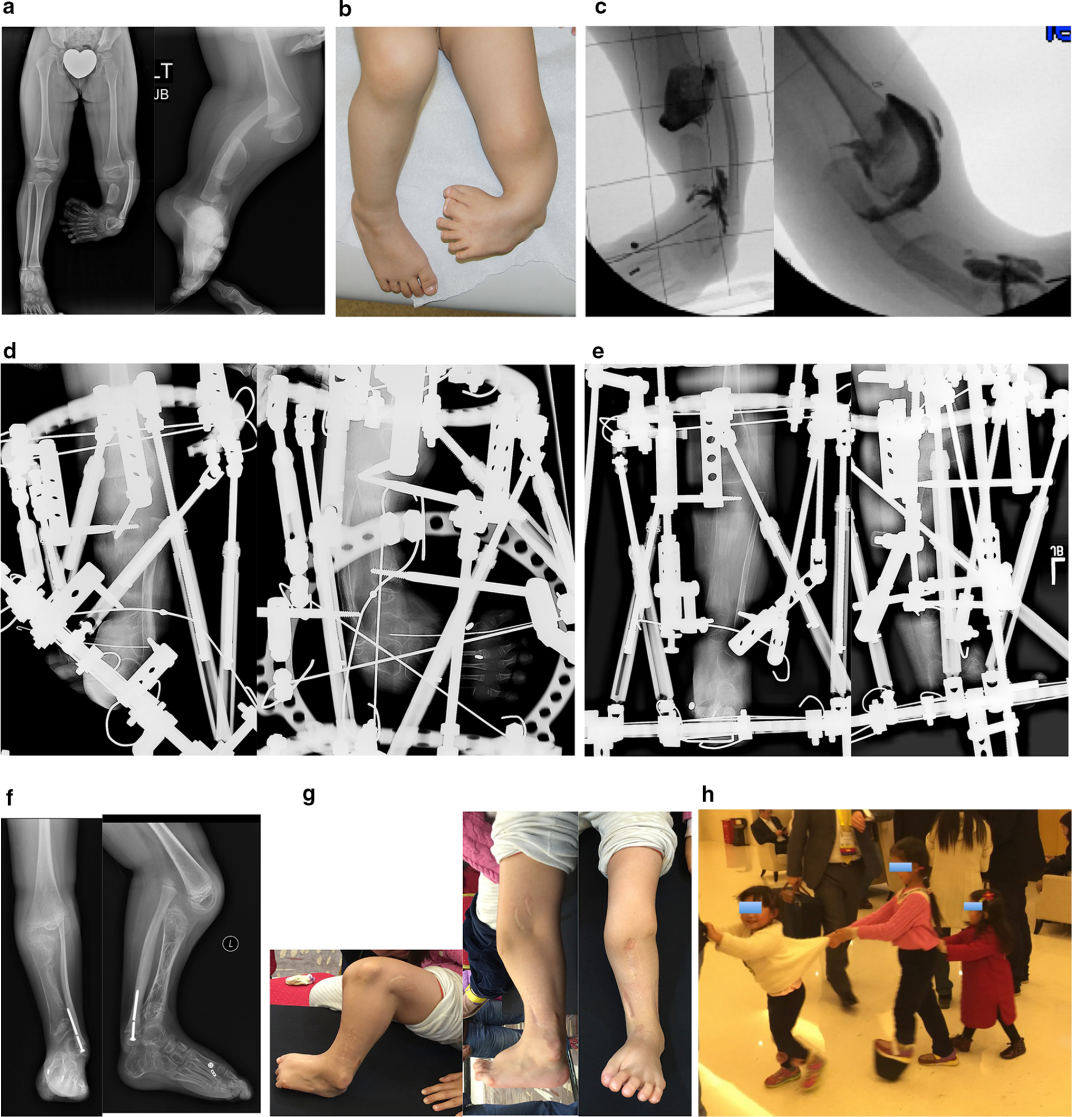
a Radiographs of a 3-year-old girl with unilateral tibial hemimelia, Paley type 2B tibial hemimelia + metatarsals/toes. The obvious delta tibia is seen with two secondary ossific nuclei. The foot has seven metatarsals and eight toes. The foot is in equino-varus. The ankle and knee joint are maloriented. The proximal fibula is relatively overgrown and sits proximal to the distal femoral physis. b Clinical photograph of same girl. c Arthrogram of knee and ankle showing joint malorientation and dysplasia. d AP (*left*) and lateral (*right*) radiographs with TSF in place and osteotomy lengthening and deformity correction of the tibia. The foot deformity correction is partially through the distal tibial reorientation. Note there is no osteotomy of the fibula and that it is only fixed to the distal tibial ring using one distal tibio–fibular wire and half pin. e AP (*left*) and lateral (*right*) radiographs following 8 cm of tibial lengthening and deformity correction with distal fibular transport. The frame was modified connecting the distal tibiofibular wire and fibular half pin to the proximal ring. This frees the ankle joint for distraction correction of the remaining foot deformity. Note that the proximal fibula has been pulled down almost to station. f Two years after removal of the external fixator the tibia is well consolidated. The fibula is already showing significant relative proximal and distal overgrowth. The distal fibular epiphysiodesis screw’s head is migrated into the epiphysis and screw shaft is broken rendering it mostly ineffective at this juncture. The foot varus deformity is slowly recurring. This will require another differential lengthening surgery in a year or two. g Clinical photos showing knee and ankle range of motion two years after lengthening. h This girl is very active despite recurrent leg length difference and mild foot deformity. Note how she plays with the other girls while she wears a shoe lift. She is the tall girl in the *middle*
